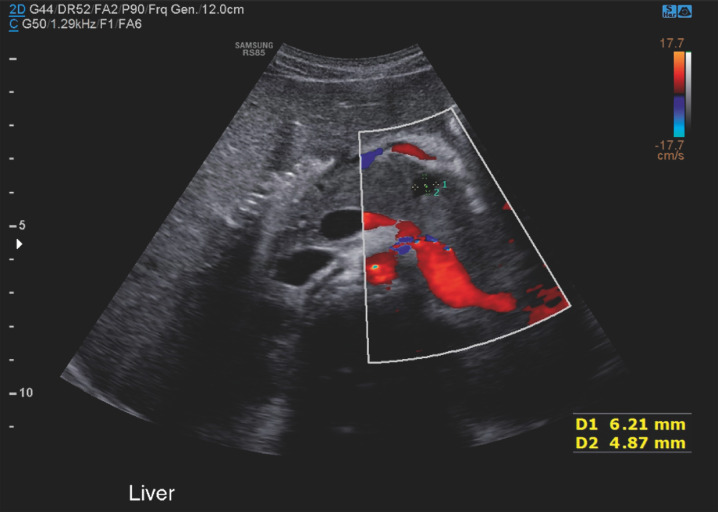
Ultrasound of the abdomen showing resolved fluid collection in the pancreas previously seen on abdominal MRI.
